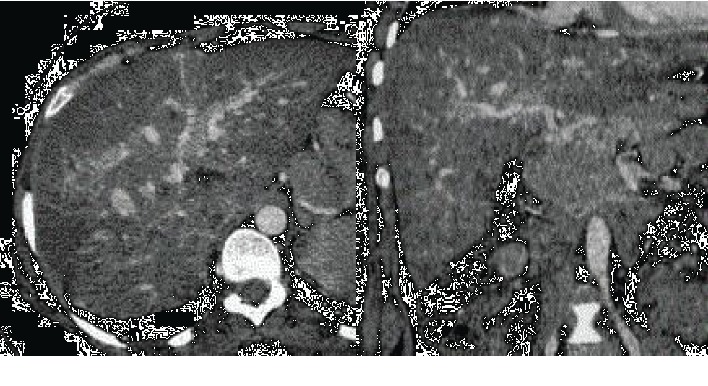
The transversal and sagittal section of the CT scan shows arteriovenous disorders in the liver.
